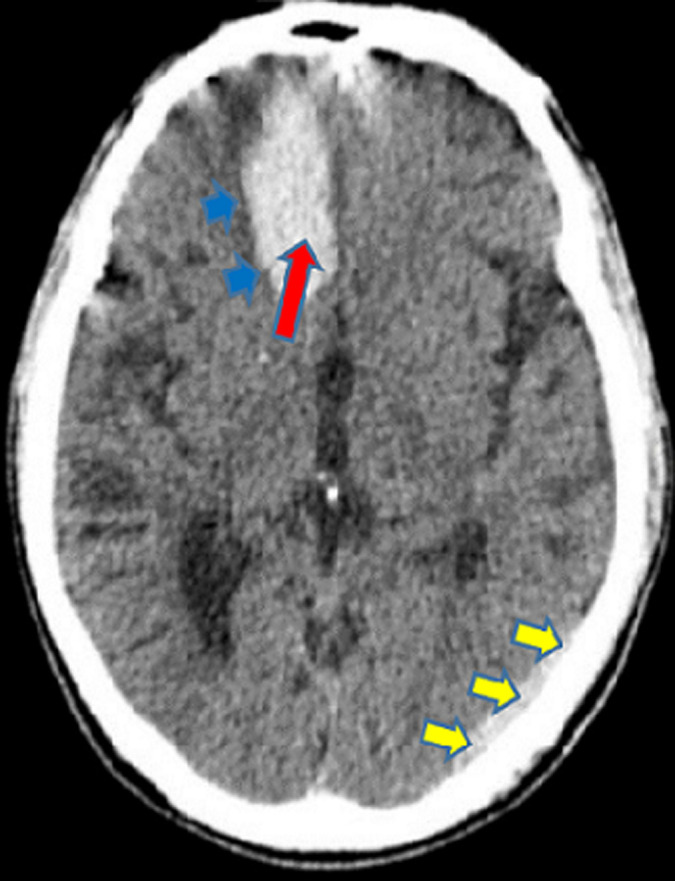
an axial non-enhanced CT scan of the head showing an acute right frontal intraparenchymal hemorrhage (red arrow), perilesional edema without a midline shift (blue short arrows), and subtle acute left occipital epidural hematoma (yellow short arrows)
